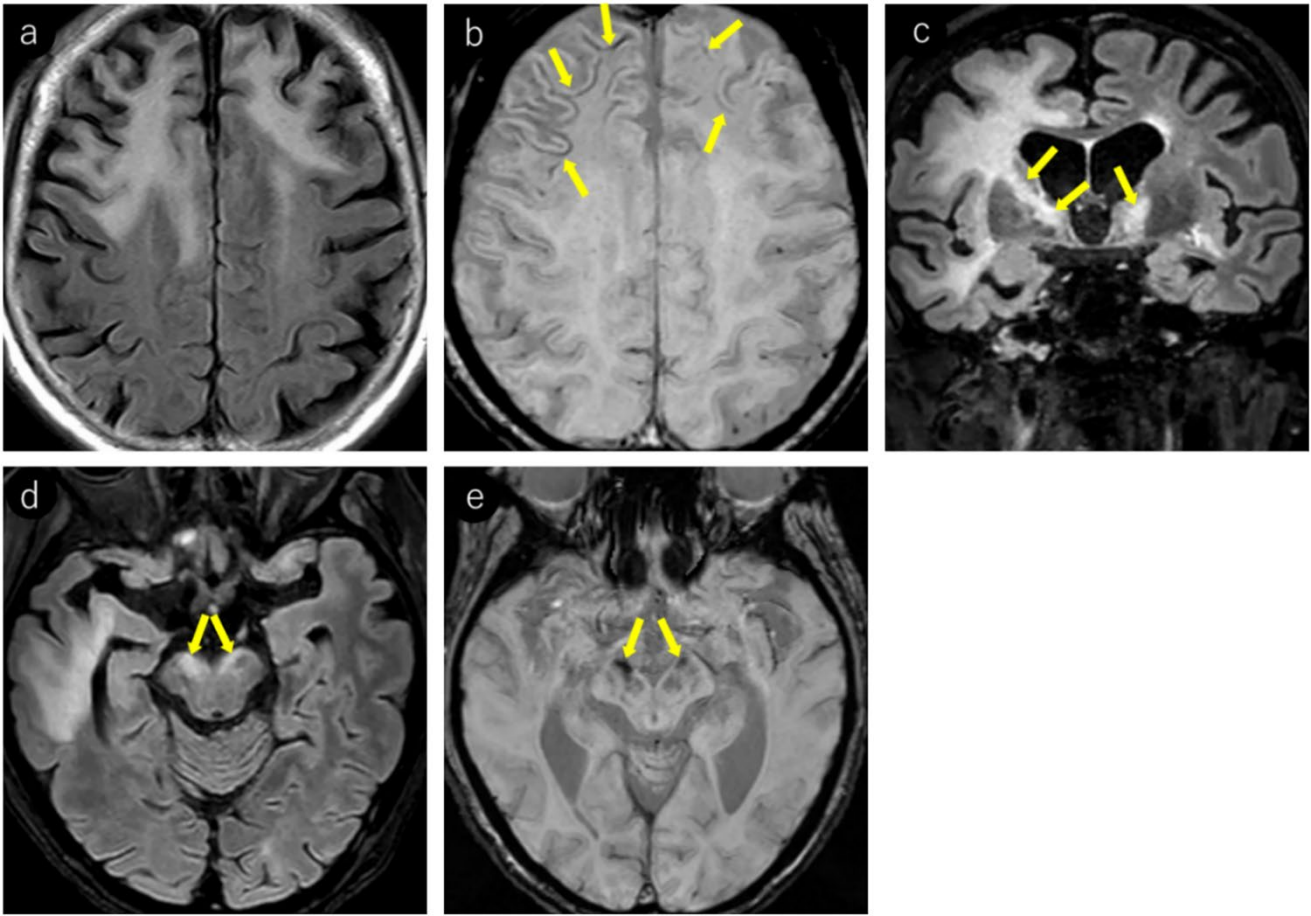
Woman in her 80 s who developed PML during chemotherapy for DLBCL. MRI findings were obtained when the patient presented with disturbed consciousness 4 months after the onset of PML. a Fluid-attenuated inversion recovery (FLAIR) imaging shows hyperintense lesions in the bilateral frontal lobes, predominantly on the right side, extending from the juxtacortical to the subcortical white matter. b Linear hypointensity is observed predominantly on the right side of the SWI in the deep cortical layers and the corticomedullary junction adjacent to the lesions (yellow arrows). c Coronal FLAIR images demonstrating right-predominant caudal lesion progression along the corticospinal tract (yellow arrows). d Bilateral hyperintense lesions are noted in the medial cerebral peduncles on FLAIR imaging, which are more prominent on the right side (yellow arrows). e Substantia nigra lesions show hypointensity on SWI with a right-sided predominance (yellow arrows)
